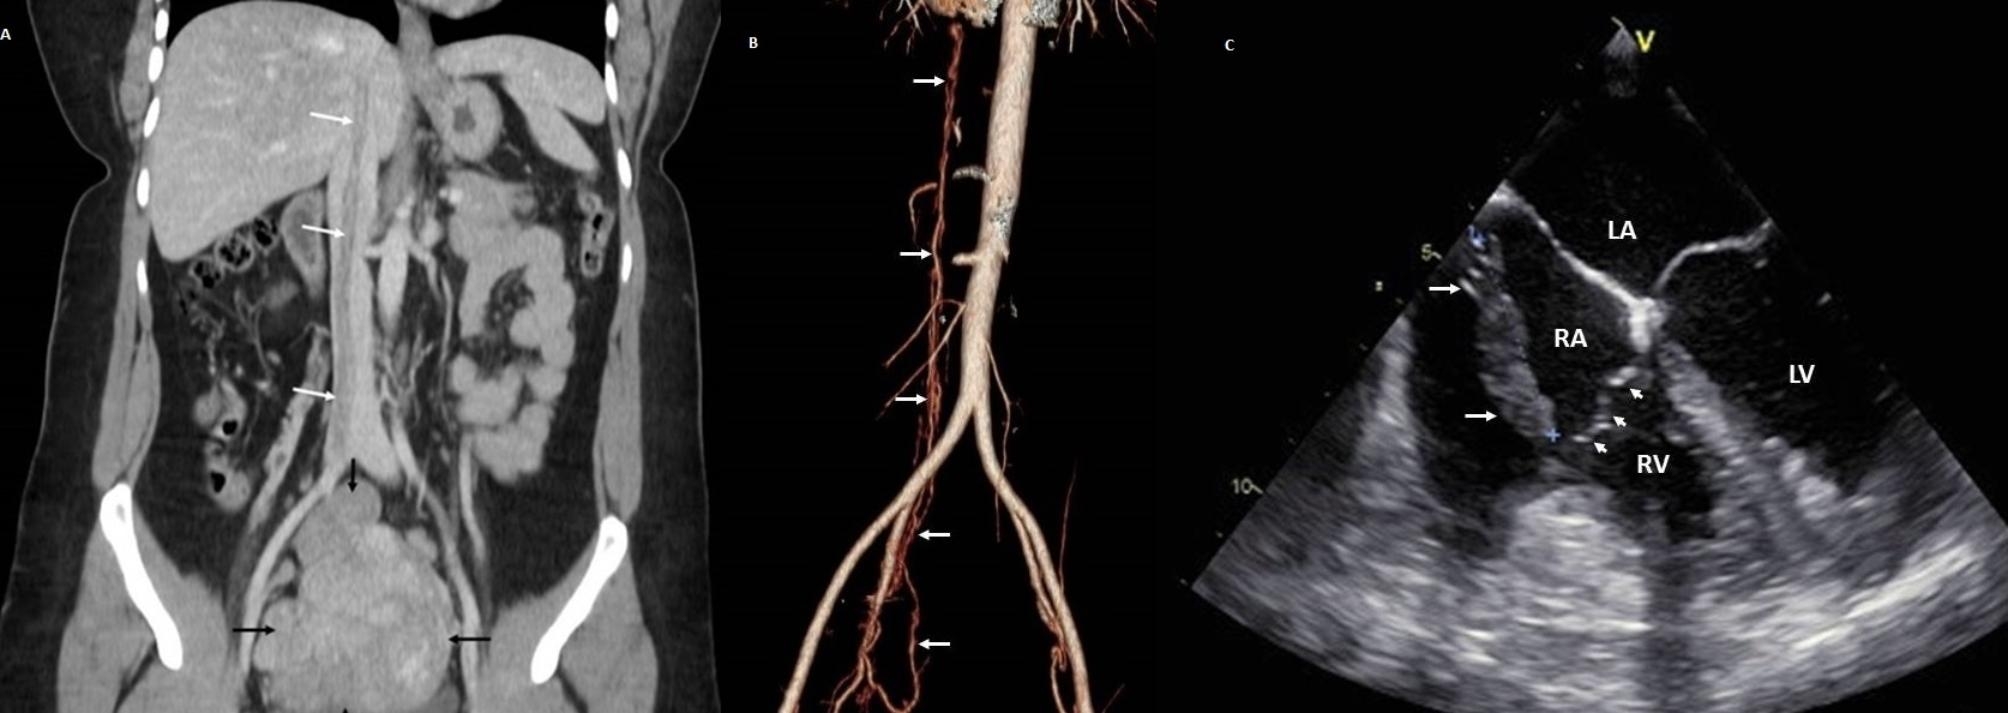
A: CT, filling defect on lower vena cava and mass on uterus. B: CT reconstruction, the whole extent of the mass is seen. C: Echocardiogram, the mass is seen within the right atrium
